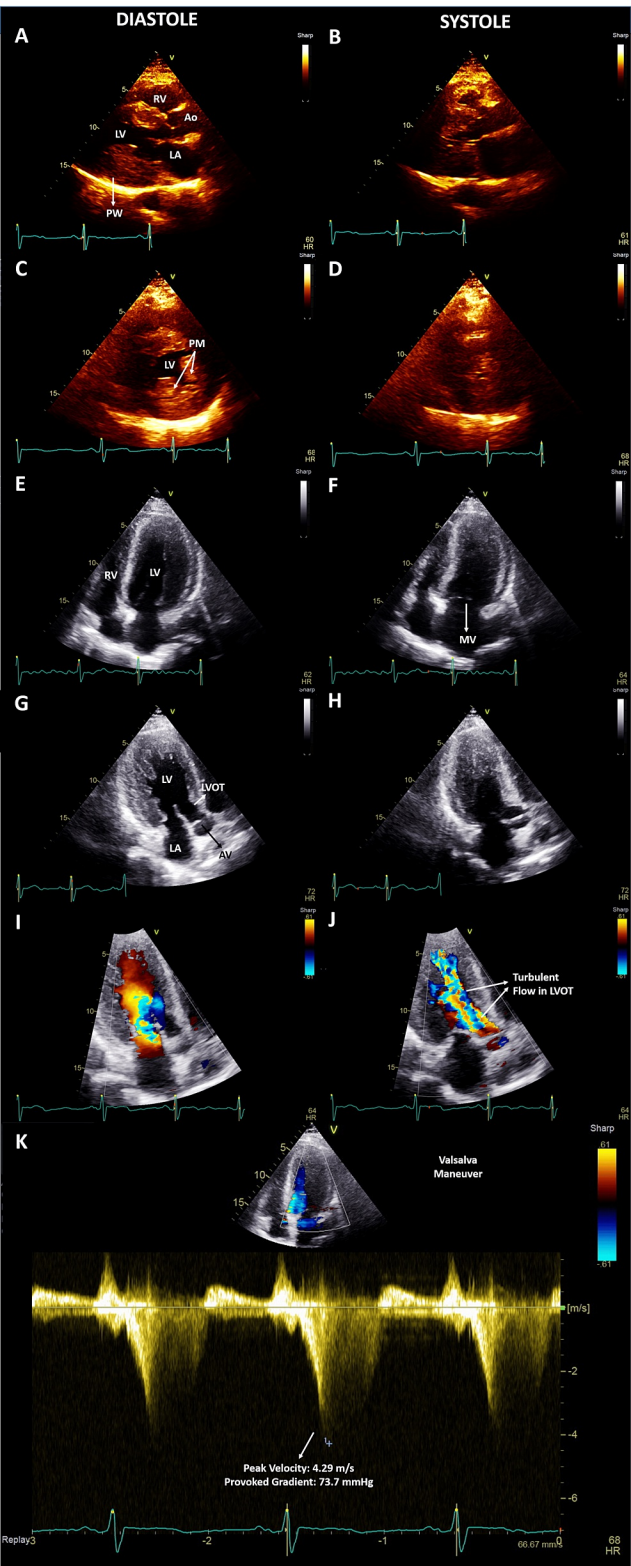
Transthoracic echocardiogram A transthoracic echocardiogram demonstrated severe left ventricular hypertrophy with thickened papillary muscles in the parasternal long (A, B), parasternal short (C, D), apical 4 chamber (E, F), and apical 3 chamber (G, H, I) views with preserved left ventricular ejection fraction and almost complete left ventricular cavity obliteration during systole. Impaired diastolic filling is present due to global left ventricular hypertrophy (not shown) along with abnormally turbulent flow in the left ventricular outflow tract during systole (J) due to septal hypertrophy without systolic anterior motion of the mitral valve. Continuous wave Doppler revealed resting and provoked gradients of 36 and 73 mmHg, respectively, across the left ventricular outflow tract (K) in the absence of aortic stenosis, confirming hypertrophic obstructive cardiomyopathy. RV - right ventricle; LV - left ventricle; Ao - aorta; LA - left atrium; PW - posterior wall; PM- papillary muscle; MV - mitral valve; AV - aortic valve; LVOT - left ventricular outflow tract
